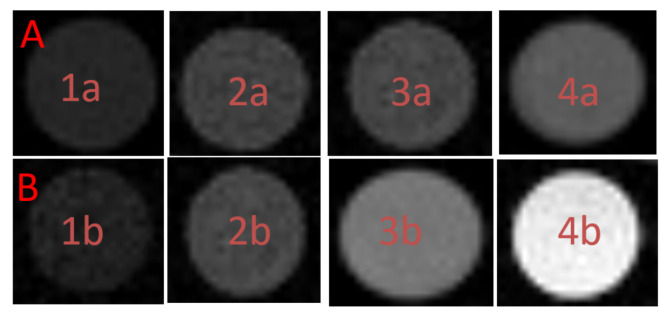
(A) An example of a spin echo *T*${}_{2}$-weighted MR image (*TR*/*TE* = 7500 ms/8 ms) of the 1AeroNPs-top (1a, 2a, 3a, 4a) with concentrations of 28 mg/mL (1a), 14 mg/mL (2a), 4.67 mg/mL (3a), and 2.8 mg/mL (4a), respectively (here the darker image of the tube indicates the shorter *T*${}_{2}$, the higher concentration of NPs); (B) An example of a *T*${}_{1}$-weighted MR image (*TR*/*TE* = 3 ms/1.5 ms) of the 1AeroNPs-top (1b, 2b, 3b, 4b) with concentrations of 28 mg/mL (1b), 14 mg/mL (2b), 4.67 mg/mL (3b), and 2.8 mg/mL (4b), respectively.
